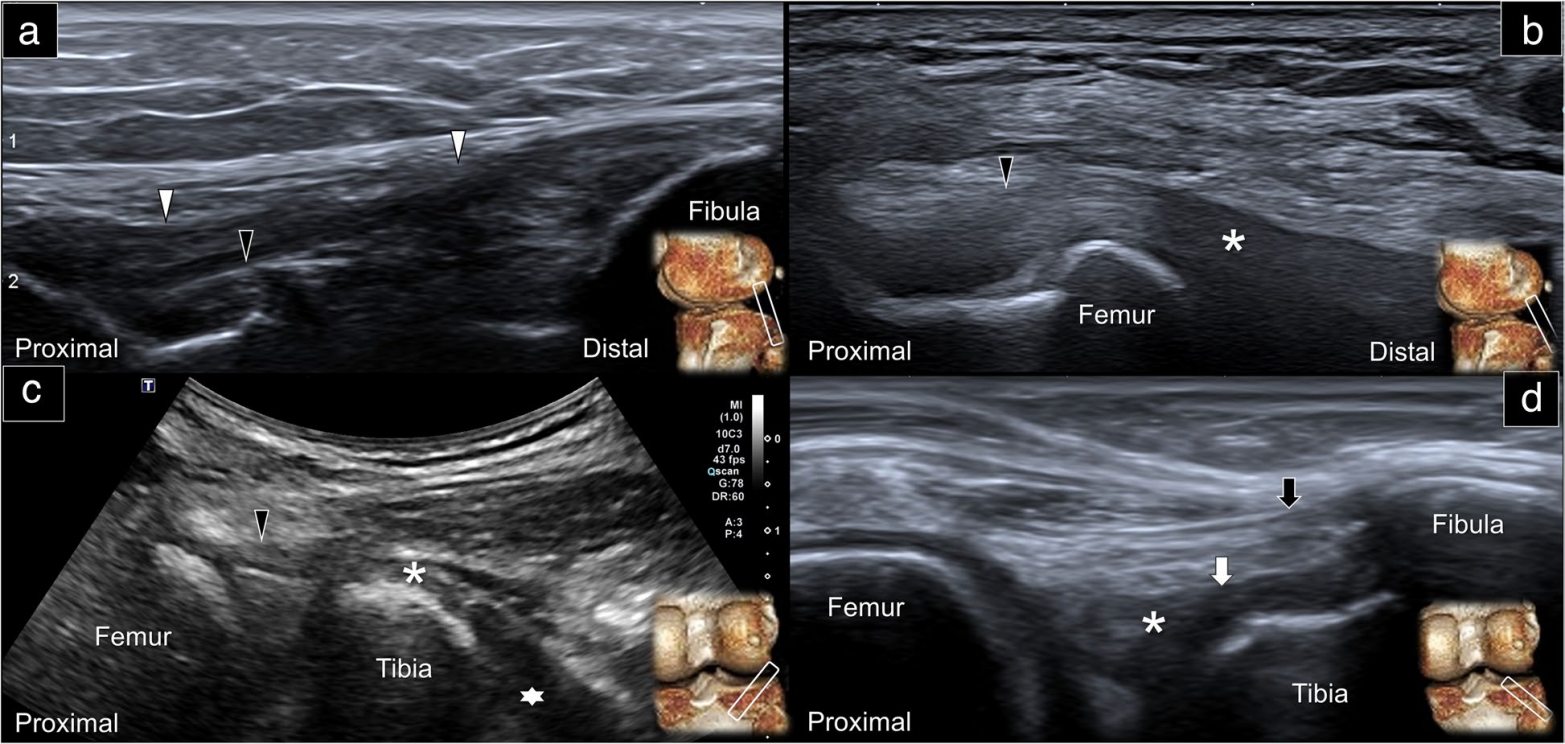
Sonographic imaging of the lateral collateral ligament, popliteus tendon (a), popliteus myotendinous junction (b), popliteus muscle (c), and popliteofibular and arcuate ligaments (d) using the long-axis approach. White arrowhead: lateral collateral ligament; black arrowhead: popliteus tendon; asterisk: popliteus myotendinous junction; star: popliteus muscle; white arrow: popliteofibular ligament; black arrow: arcuate ligament; white rectangles: transducer positions
